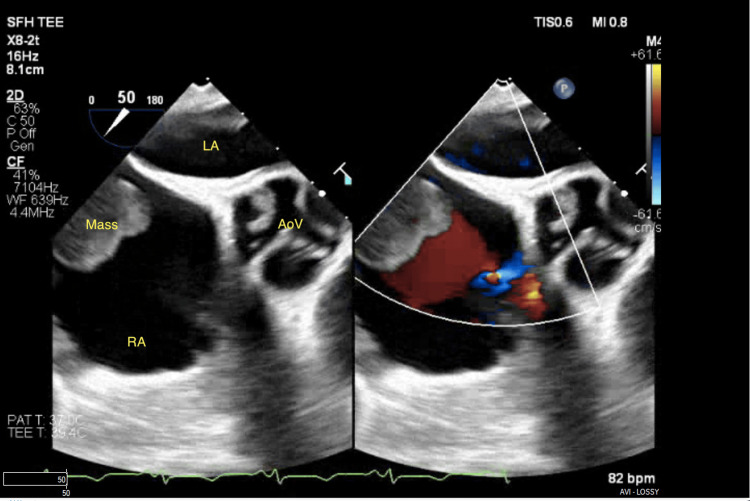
Parasternal short-axis view of the right atrial mass LA: left atrium; RA: right atrium; AoV: aortic valve
